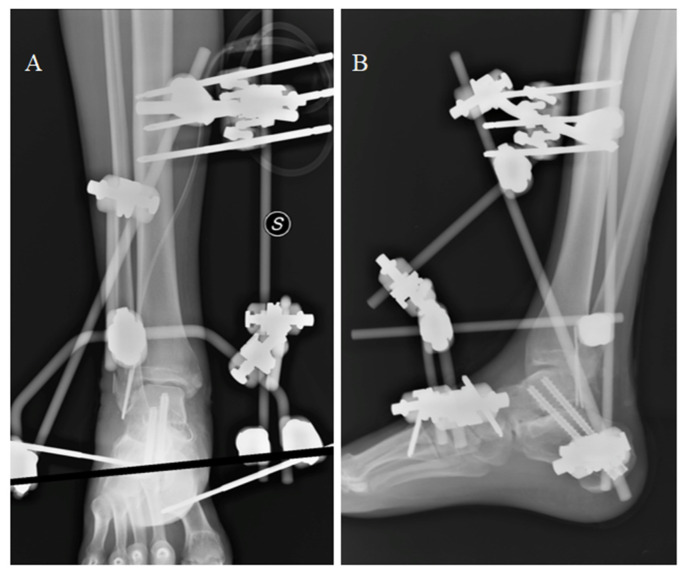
Talar reimplantation with subtalar arthrodesis: anteroposterior (A) and latero-lateral (B) X-ray.
